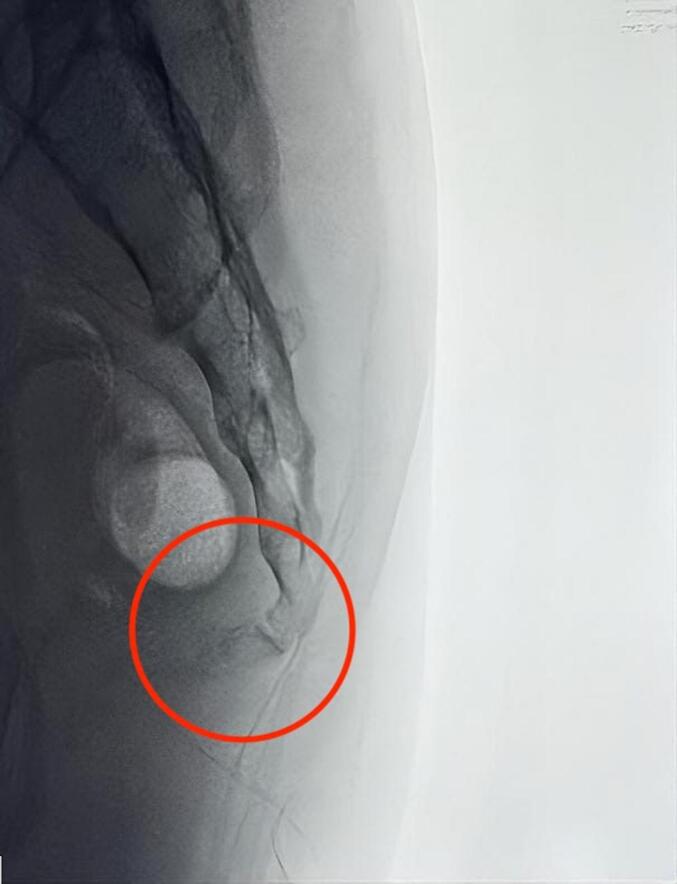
Lateral view sacrococcygeal X-ray at three months postoperative.
